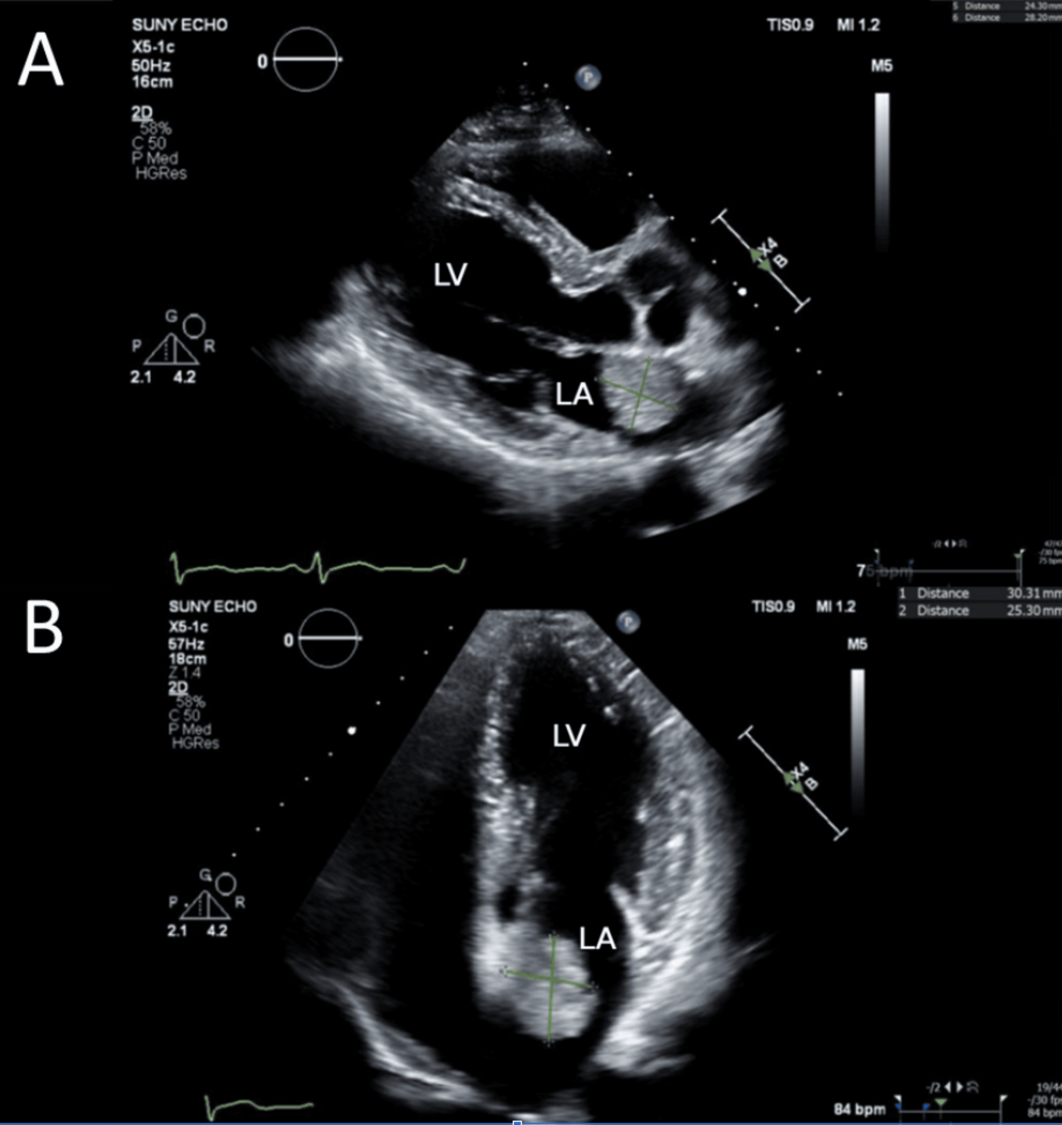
(A) Echocardiogram parasternal long axis view showing mass in the left atrium attached to the interatrial septum measuring 3.0 x 2.5 cm. (B) Echocardiogram apical four-chamber view showing redemonstration of the left atrial mass attached to the left atrial wall. LA: left atrium; LV: left ventricle.
